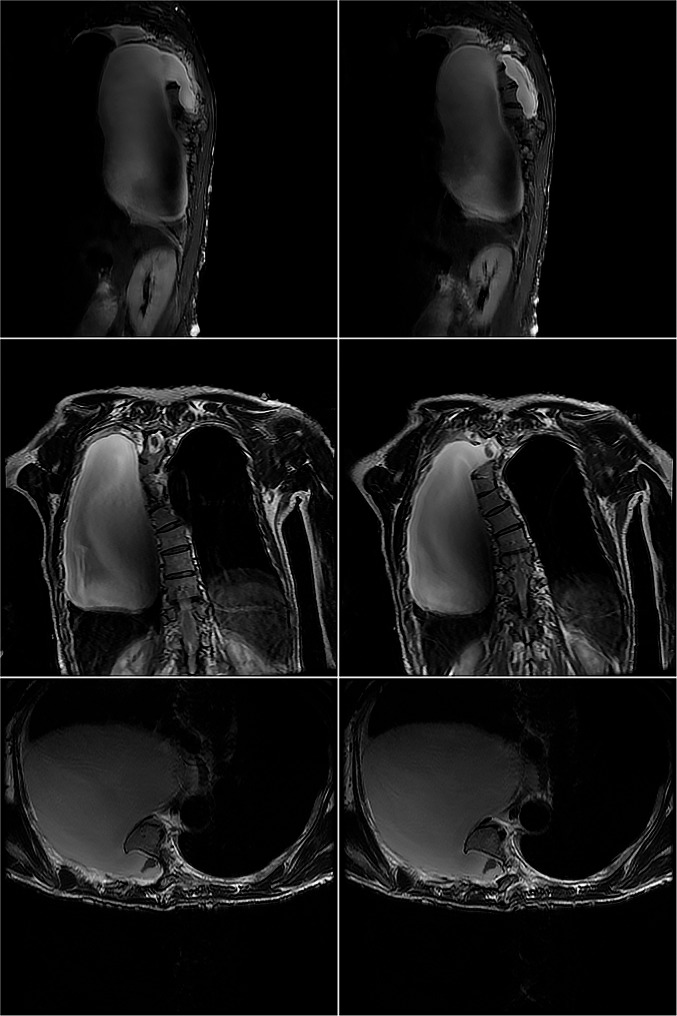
MRI revealed: 1) discontinuity between the T4-T8 vertebrae in both sagittal and horizontal axial views, 2) protrusion of the thoracic vertebrae towards the right, 3) communication between the right pleural effusion and the spinal canal. Most importantly, the MRI revealed that the ‘pleural fluid’ had similar intensity to CSF, indicating the presence of an intrathoracic meningocele
